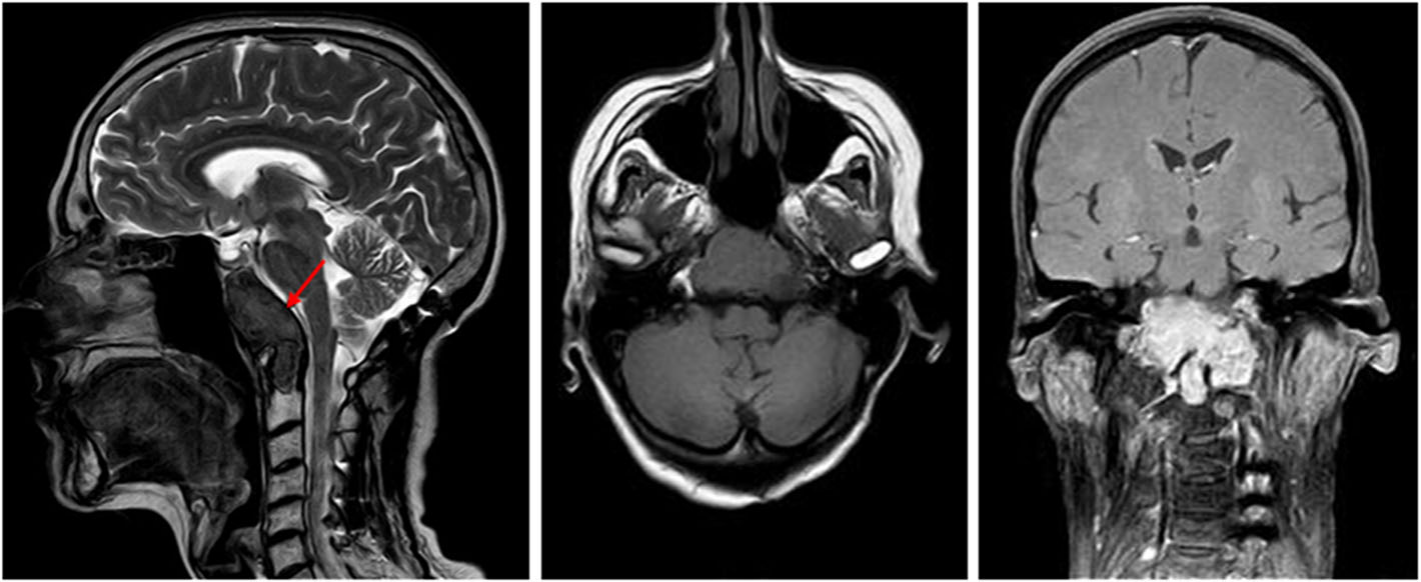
MRI images. Sagittal, axial and coronal magnetic resonance images of patient 1, depicting the large and recurrent giant cell tumour originating from the clivus (indicated by the red arrow in the sagittal view), with a C1 vertebral infiltration
